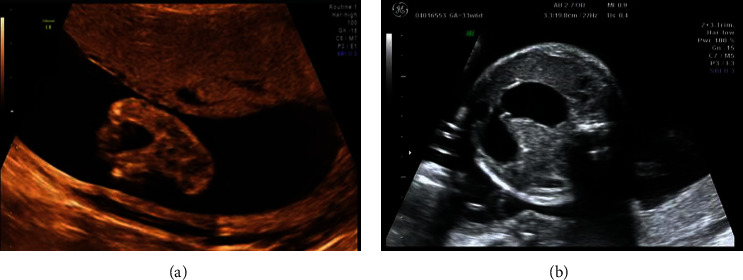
Two-dimensional ultrasonic imaging features of malformed fetuses ((a) two-dimensional ultrasonography shows a “crab pincer” splitting of one foot; (b) two-dimensional ultrasonography shows severe dilation to the stomach and duodenum near the obstruction, with fluid retention and double bubble signs).
